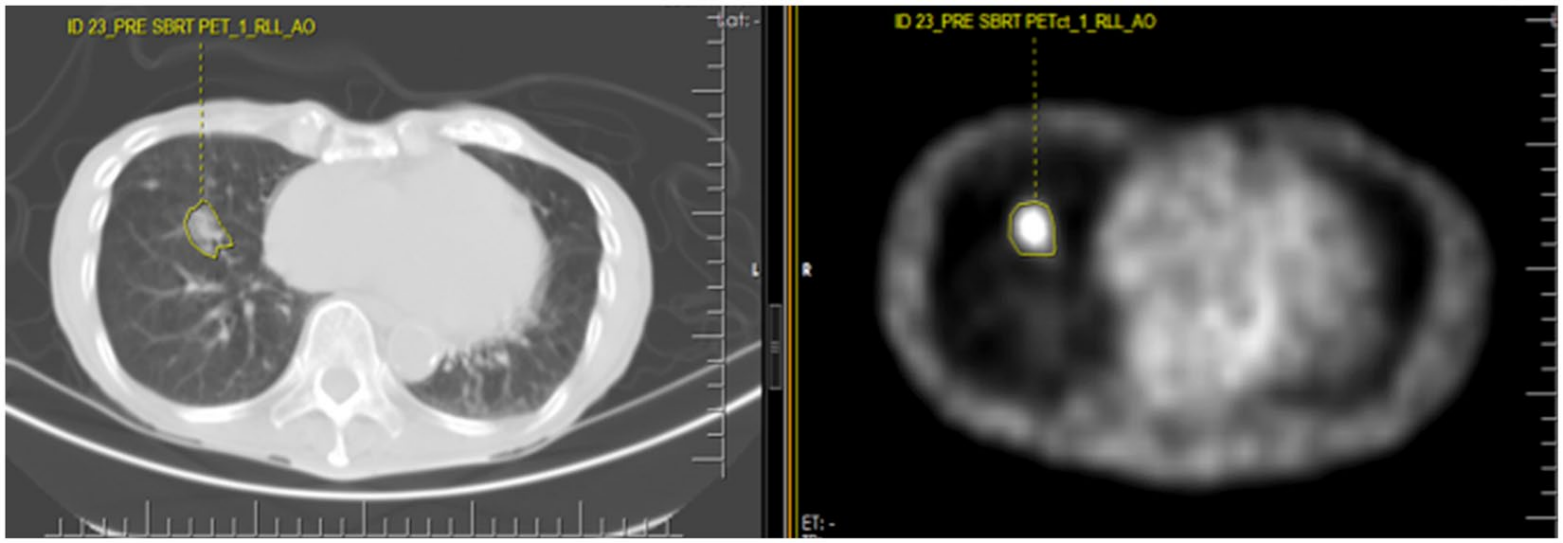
Screenshot of the texture analysis software applied on a staging PET/CT study for a NSCLC patient before SBRT therapy. On the left is the CT image and on the right, is the PET image of the PET/CT at the exact same level. The manual contouring of the right lower lobe tumor on both images is noted. There was an event of distant metastasis and death. SUVmax = 1.9. The significantly low SUVmax failed to predict the poor clinical outcome as evidenced by the development of distant metastasis and ultimately death.
